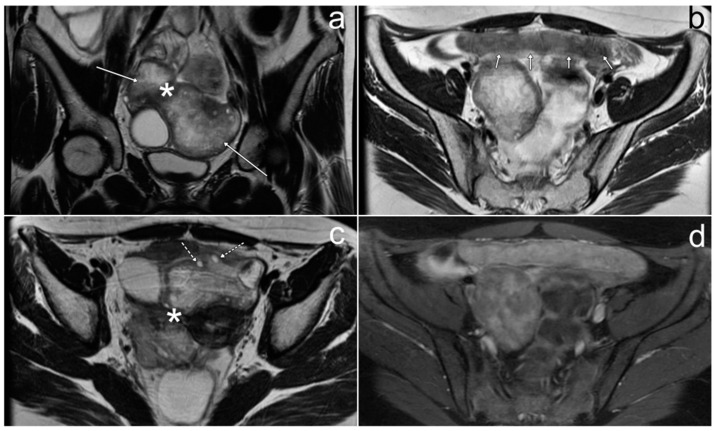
The MRI showing diffuse large B-cell lymphoma of both ovaries and peritoneal infiltrates (a–d). Enlarged ovaries touching each other ((a,c), long arrows, asterisks). Ovarian follicles at the periphery of masses ((b), dotted arrows). Peritoneal infiltration (b, short arrows). (a–c)—FSE/T2; (d)—post-contrast LAVA/T1 WATER.
